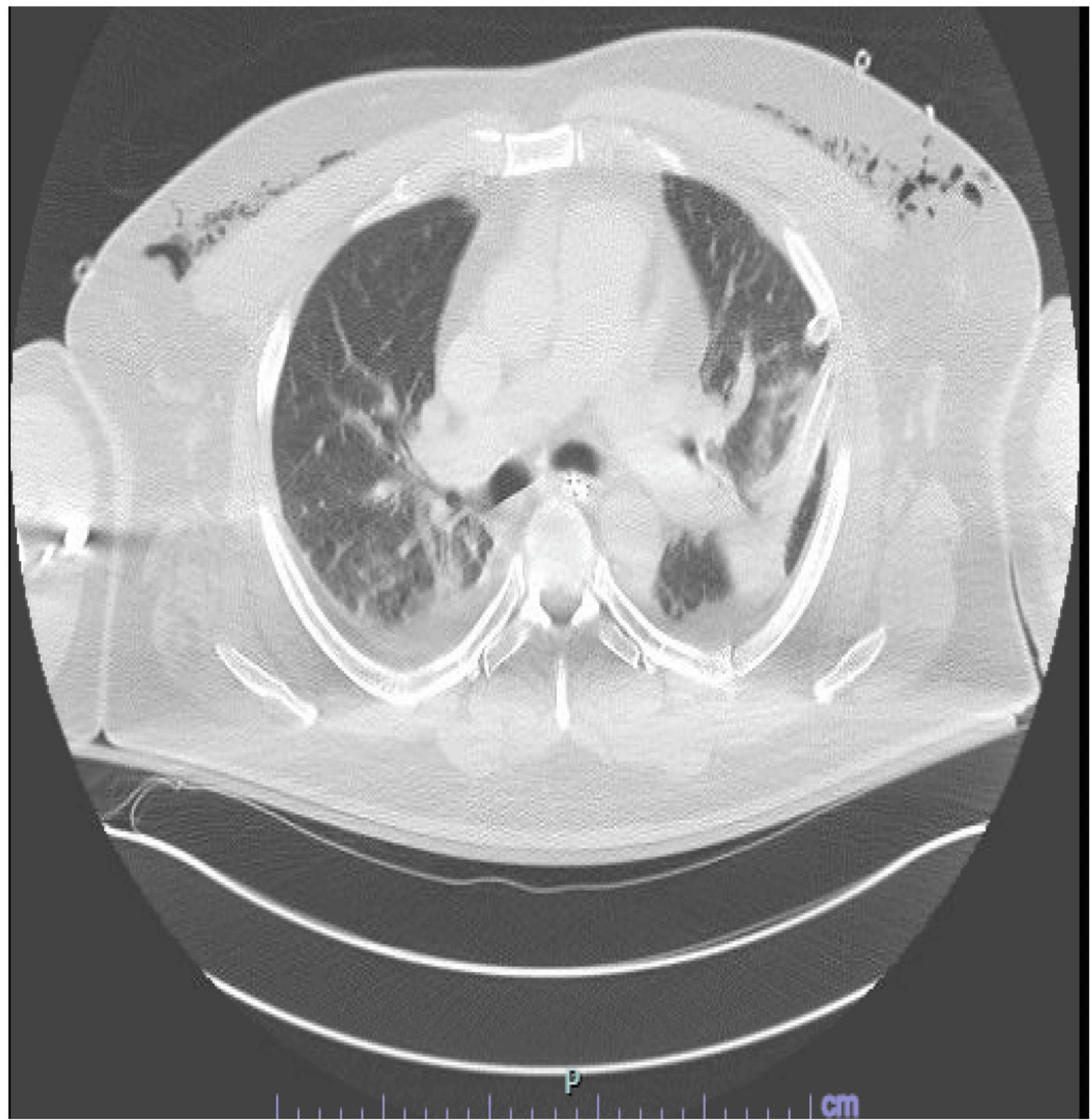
A repeat chest CT performed on postoperative day 1 following NPWT placement, showing improvement in subcutaneous emphysema and moderate pneumomediastinum. Rib fractures and small amounts of right and left pleural fluid, as observed on prior CT imaging, remained unchanged. NPWT: negative pressure wound vacuum.
